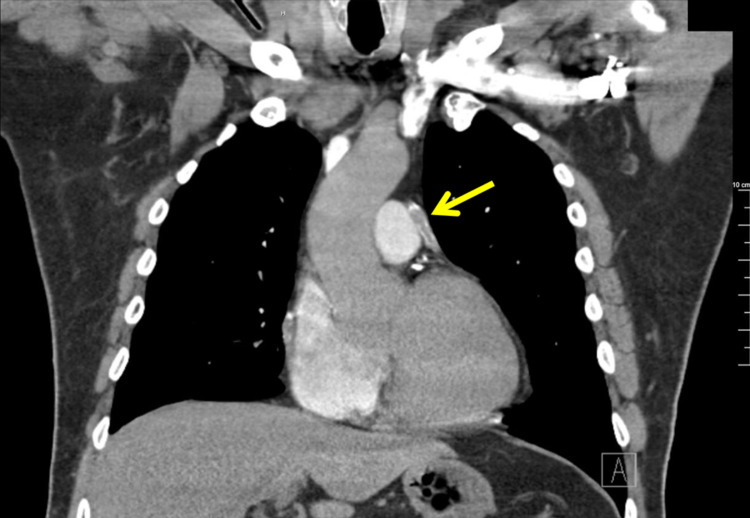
Computed tomography angiogram of the chest demonstrating calcific disease in the SVG to the first diagonal branch of the LAD coronary artery. SVG, saphenous vein graft; LAD, left anterior descending
